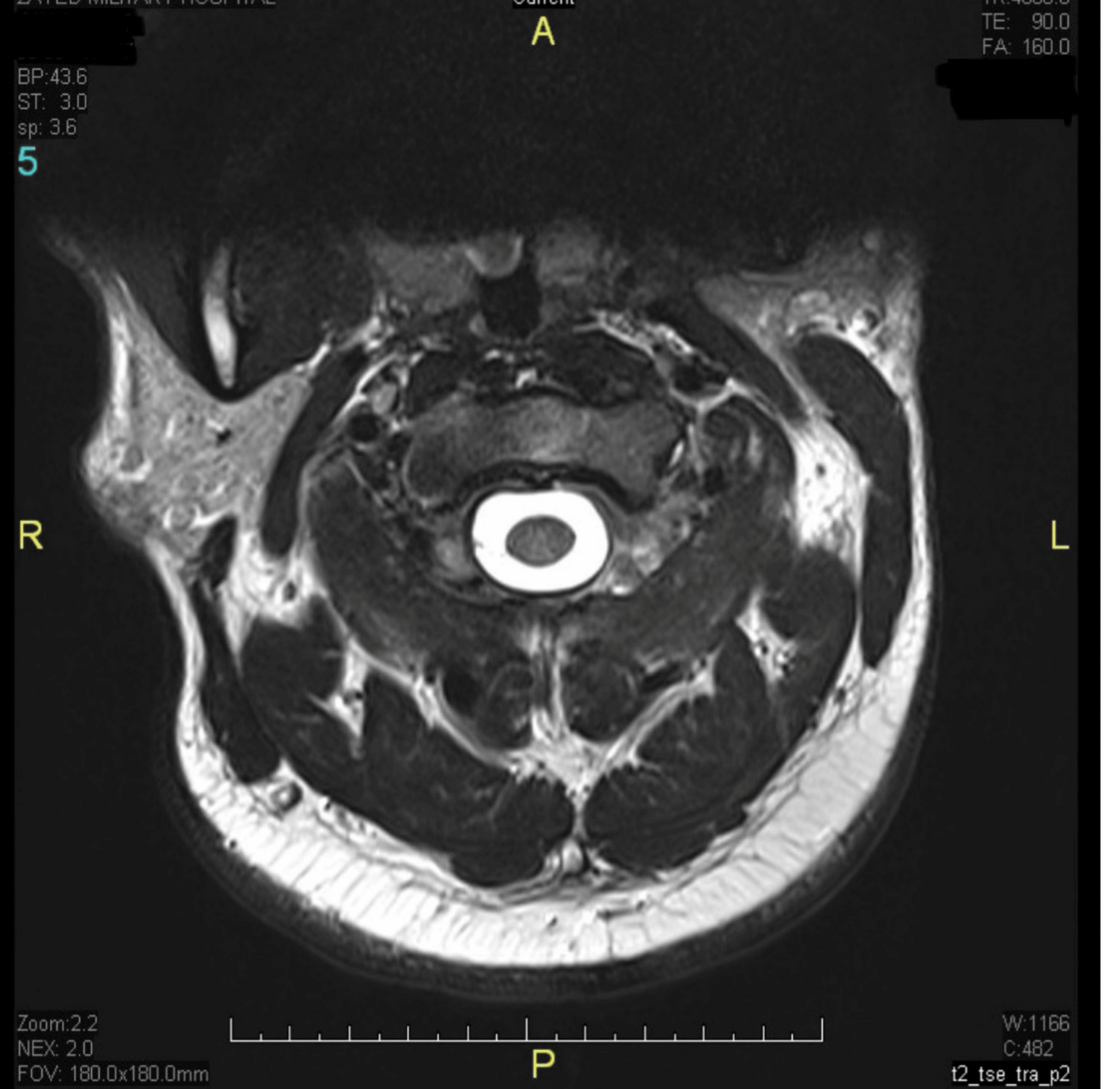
Follow-Up Axial T2-Weighted MRI Demonstrating Resolution of Cord Signal Abnormalities Repeat axial T2-weighted MRI demonstrates resolution of the prior dorsal column hyperintensity (compare with Figure 1). The spinal cord signal has normalized, consistent with radiological recovery after treatment.
